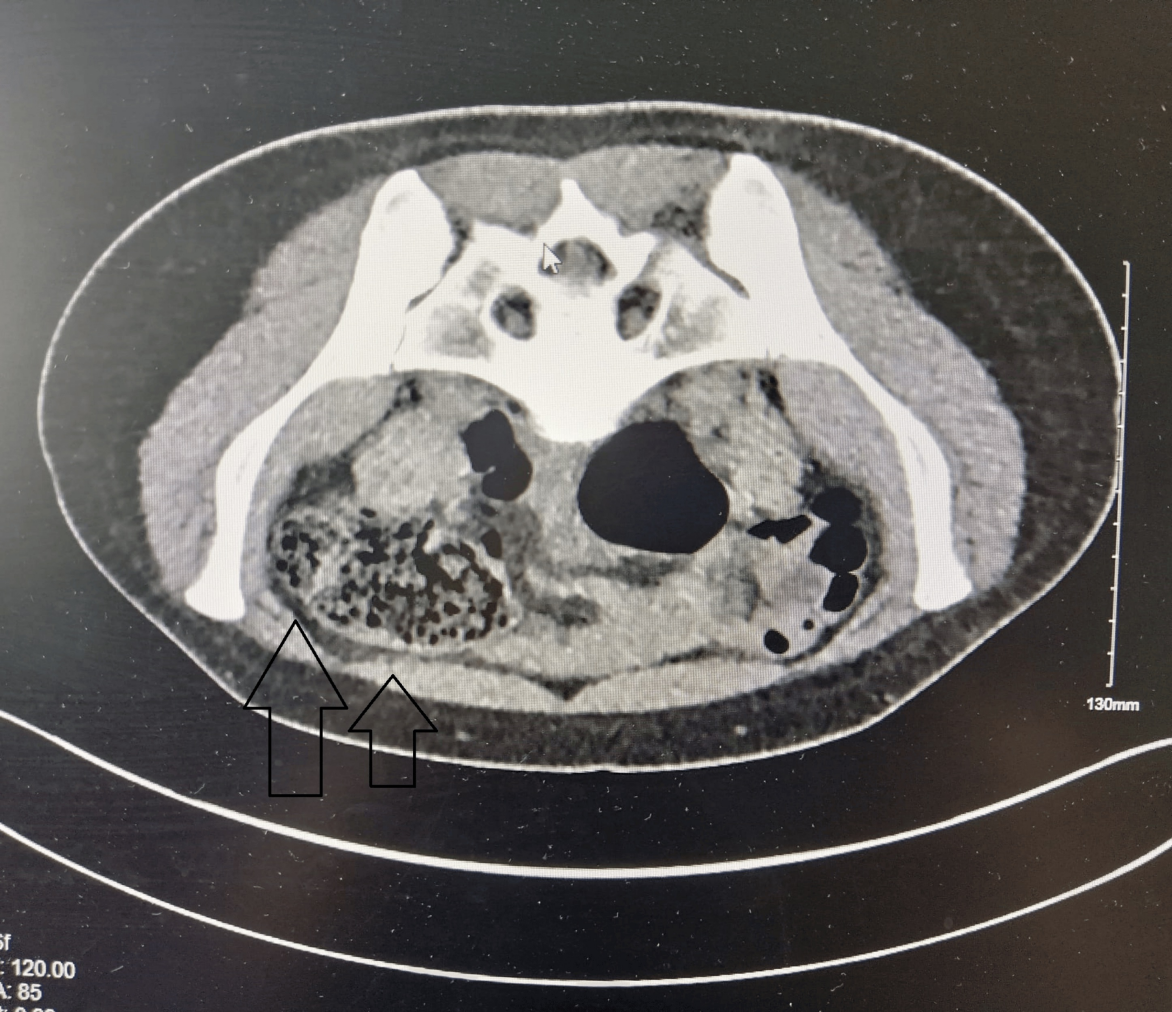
Axial CT-KUB (kidney, ureters, bladder) showing a large right adnexal cyst adjacent to the right ovary The image demonstrates a well-circumscribed, low-attenuation cystic lesion occupying the right adnexal region.
